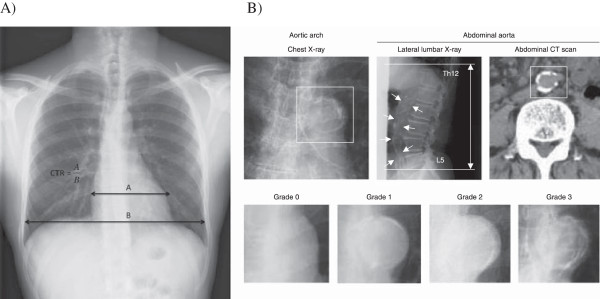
Clinical assessment of cardiothoracic ratio and aortic arch calcification. A) Measurement of cardiothoracic ratio (CTR). CTR is equal to the maximal cardiac width divided by thoracic width, as shown. B) Assessment of aortic calcification using chest x-ray (lateral lumbar and abdominal CT for comparison with calcification enclosed by white boxes), with examples of grade 0–3 shown. Permission pending for reproduction of this image [12].
